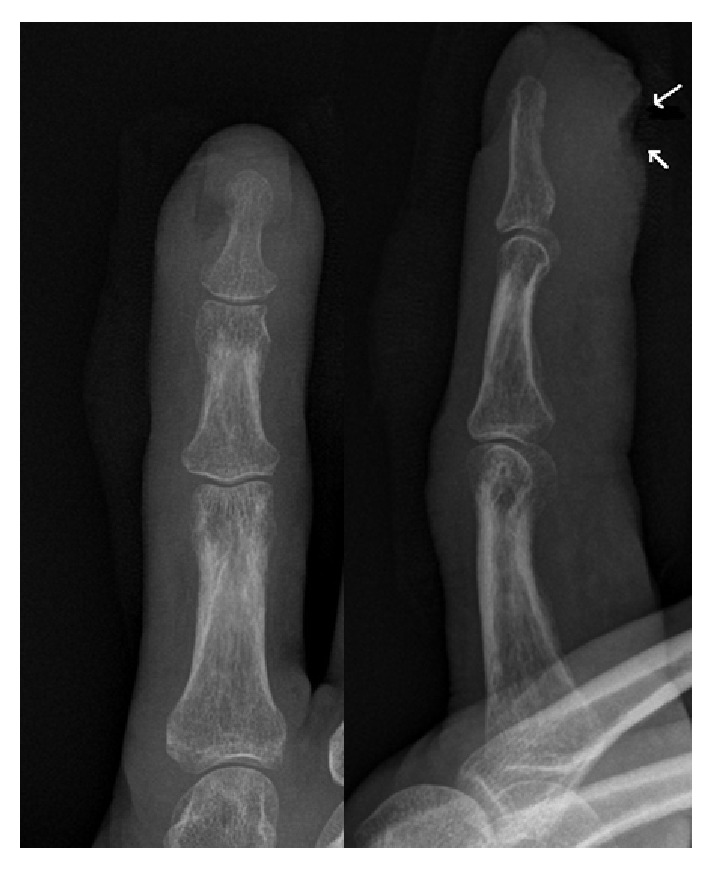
Preoperative anteroposterior and lateral radiographs of the index finger. Arrows: necrotic area in the soft tissues.
